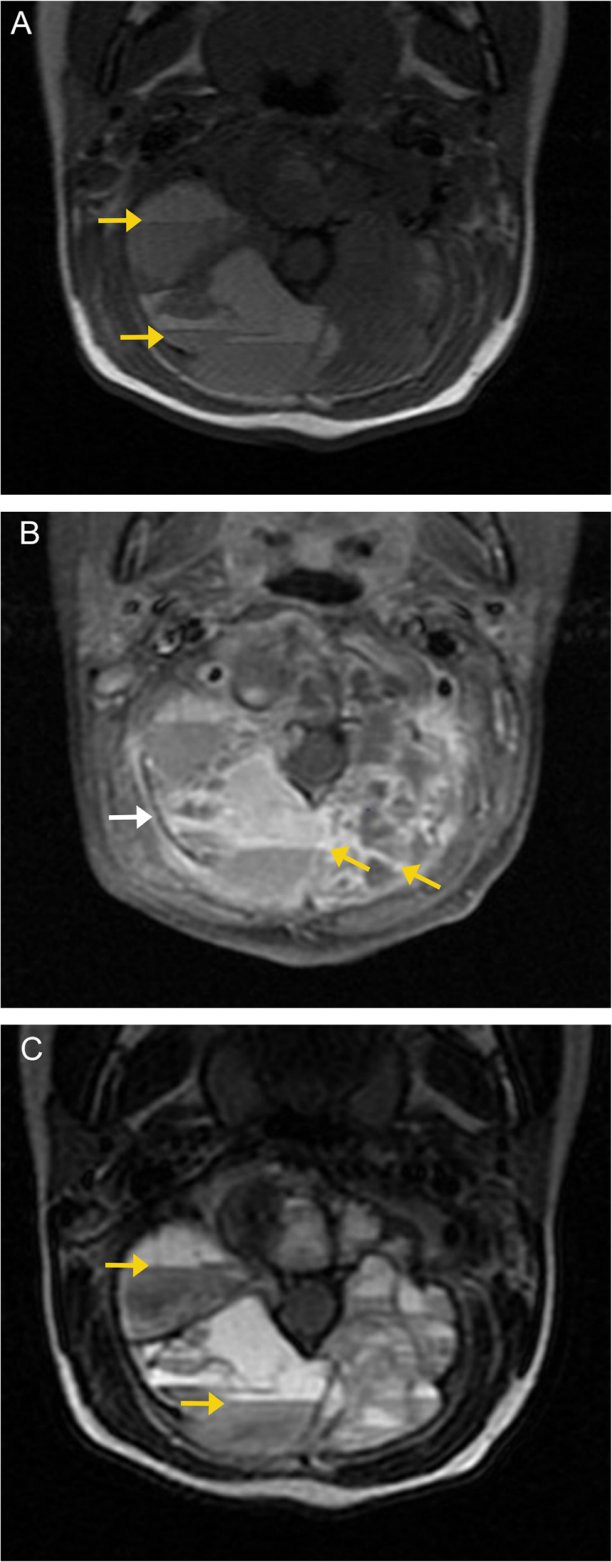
Aneurysmal bone cyst. MRI imaging in the same patient as Fig 9. Pre-contrast axial T1-weighted image (a) confirms fluid-fluid levels within the lesion (orange arrows), indicating hemorrhage with sedimentation of varying stages of blood products. Post-contrast axial T1-weighted image (b) demonstrates enhancement of the septa within the lesion (orange arrows). Note a partially hypointense rim, representing cortex (white arrow). Axial T2-weighted image (c) re-demonstrates a destructive lesion with fluid-fluid levels (orange arrows); varying signal intensity corresponds to differing stages of blood product degradation
